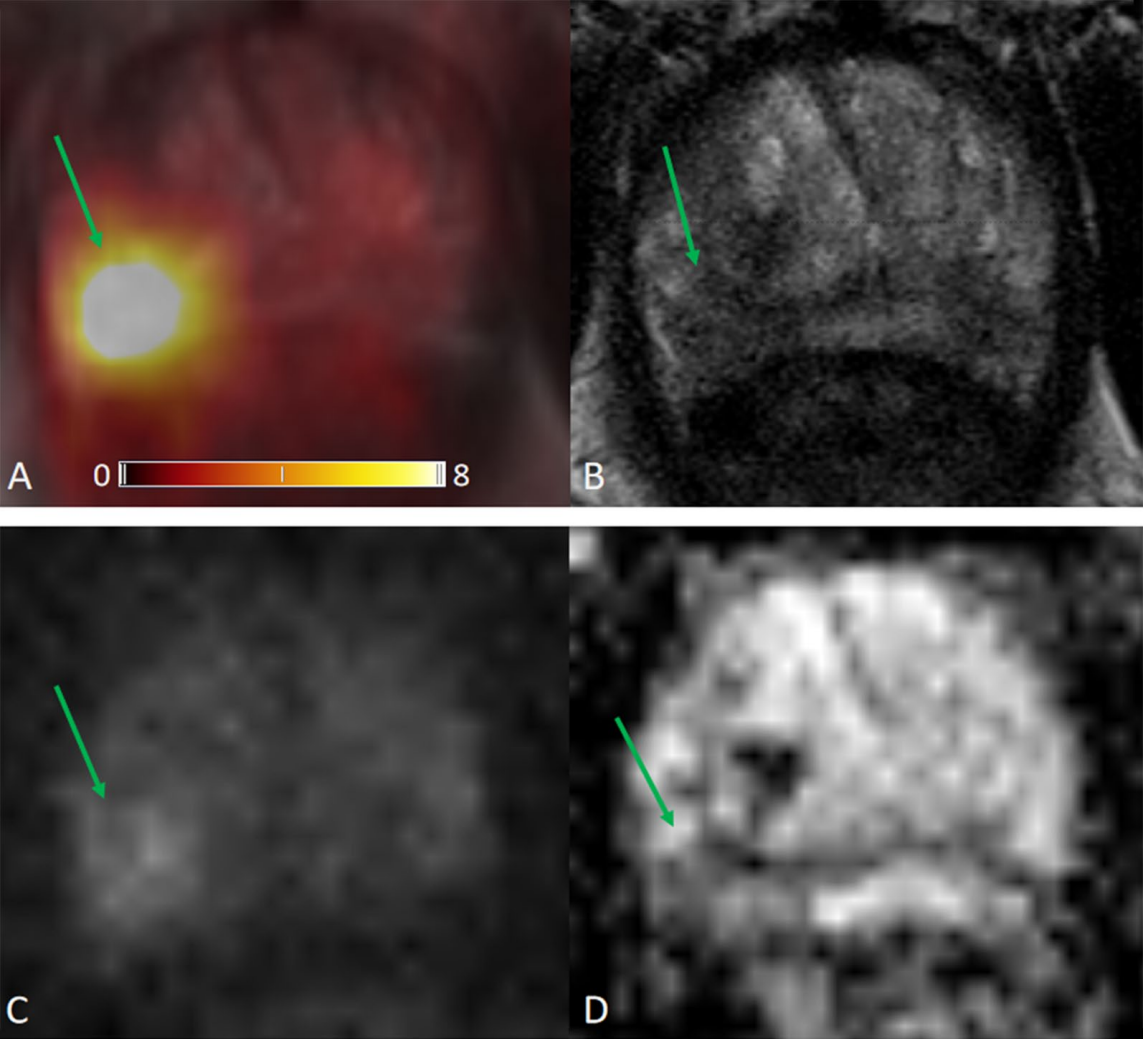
[^68^Ga]Ga-PSMA PET/MRI images obtained in a 76-year-old man, PSA 9.50 ng/mL. a [^68^Ga]Ga-PSMA PET/MRI; b T2WI; c DWI, *b* value 1000 s/mm^2^; d ADC. This example showed MRI-positive lesions, PI-RADS 4, with apparent PSMA uptake in [^68^Ga]Ga-PSMA-11 PET.a MRI-negative lesions, a typical encapsulated nodule with unapparent radiotracer uptake in [^68^Ga]Ga-PSMA-11 PET. SUVmax 13.6, right PZ, background SUVmax 1.5, LBR 9.1
